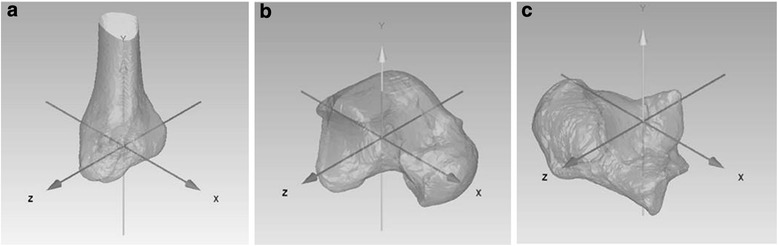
An example of the geometric bone model. (a: tibia, b: talus, c: calcaneus) After geometric bone models of the tibia and talus/calcaneus were created from computed tomography scans, 3D bone model anatomical coordinate systems were set
